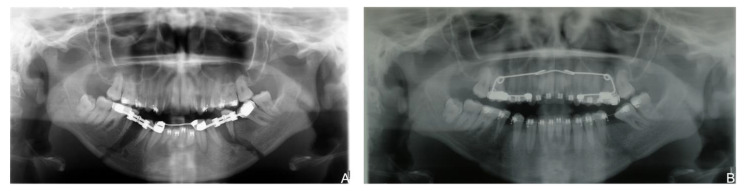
Orthopantomography immediately after the active period of distraction (A) and with six months consolidation period (B).
